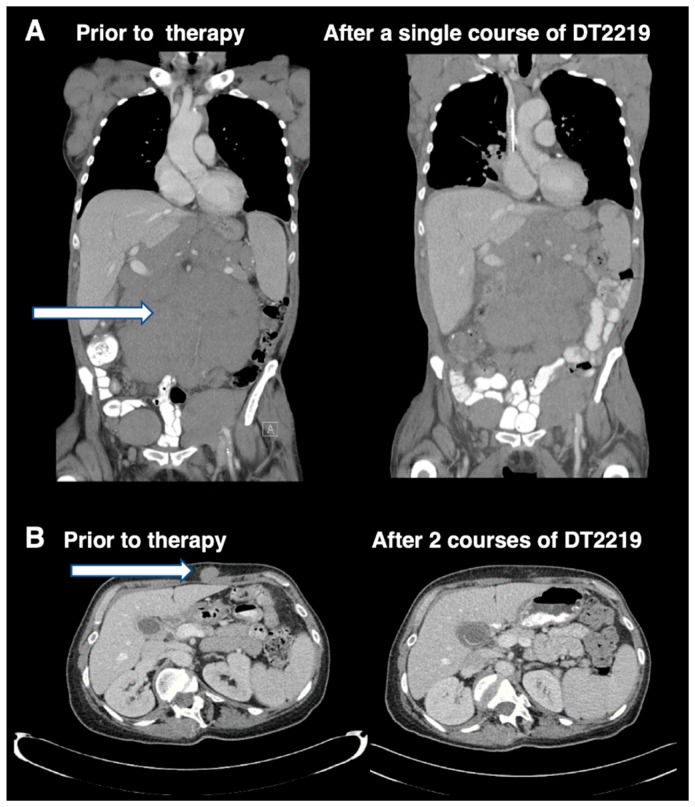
Imaging studies in patients attaining objective phase 1 study responses shown with permission of the AACR and reprinted from reference 10. Figure 1A shows an abdominal CT imaging of a 77 year-old patient with rituximab and chemotherapy-refractory CLL treated with a single course of DT2219 at dose level 40 mg/kg every other day, 4 doses before and at day 28 after therapy. A 40% reduction in the abdominal tumor mass was observed. Figure 1B shows CT images of a 53-year-old female with CD22+CD19+ relapsed, chemotherapy-refractory, marginal zone lymphoma. The patient was treated at dose level 60 mg/kg/day QOD. The patient received a second treatment course 8 weeks later, which resulted in complete resolution of the tumor mass. CT images were taken before therapy and after the second course of DT2219. Tumor mass is indicated by arrows. For greater detail, see reference 10. The image was taken at the University of Minnesota Medical Center and published with informed consent.
